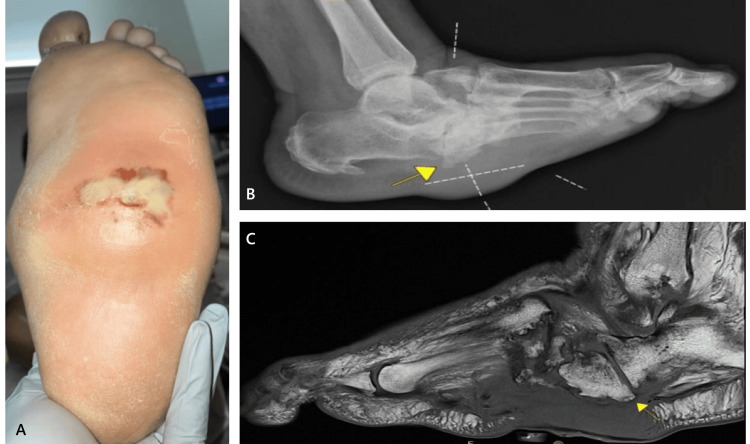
Preoperative clinical and imaging assessment. (A) Preoperative view of the non-healing plantar midfoot ulcer on the left foot, demonstrating a defect with yellow fibrinous exudate and surrounding erythematous tissue. (B) Pre-operative non-weight-bearing lateral radiograph of the left foot demonstrating chronic neuropathic midfoot collapse with a plantar exostosis (arrow) underlying the ulcer site. Associated soft tissue swelling is present. (C) T1-weighted sagittal MRI view of the left foot demonstrating inflammatory fluid accumulation at the plantar midfoot without evidence of progressive osteomyelitis. Additional findings include neuropathic arthropathy with subluxation/dislocation, cortical irregularity at the midfoot joints, and midfoot sag (arrow), consistent with advanced CNO. CNO: Charcot neuropathic osteoarthropathy
